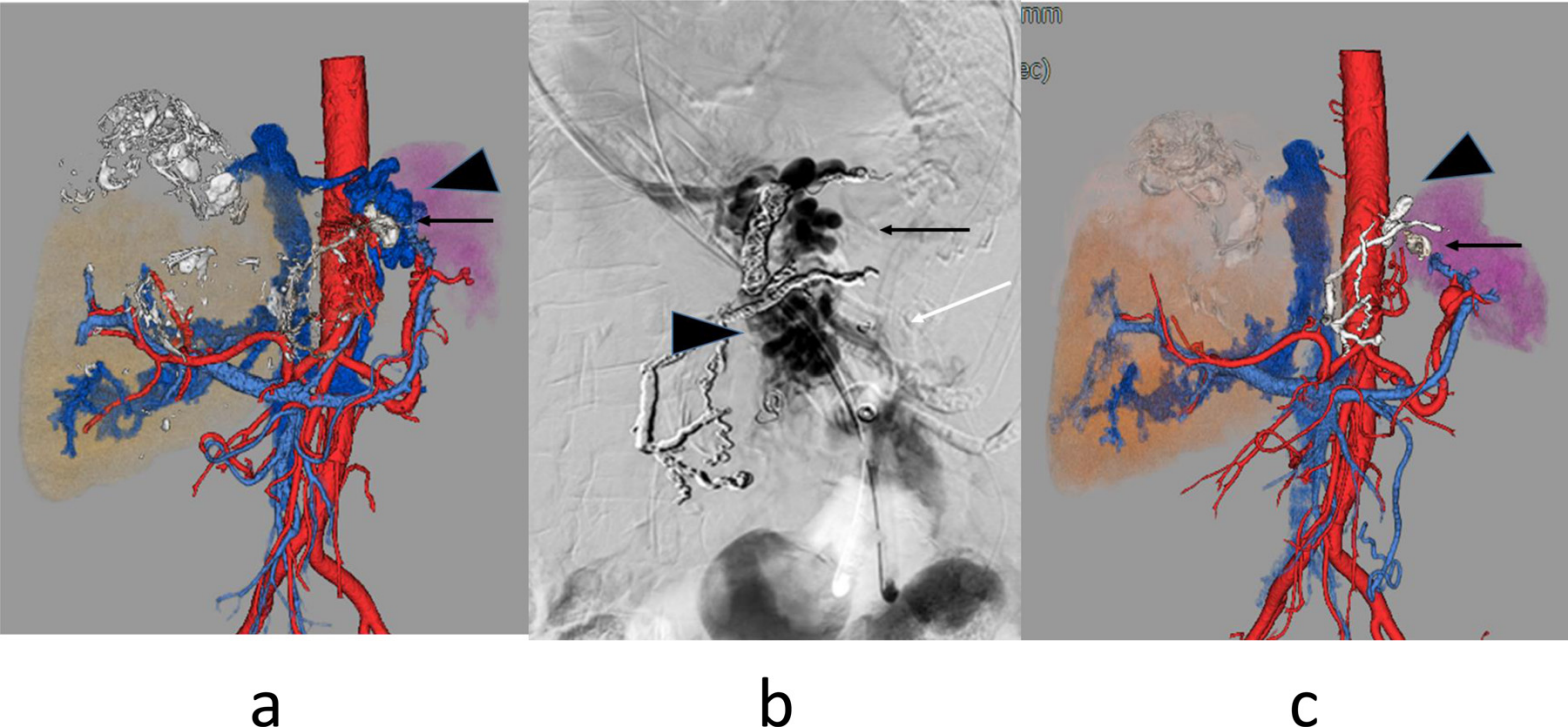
(a). 3D-CT reconstruction image after the emergency hybrid procedure in case 2 shows NBCA-Lipiodol (arrow), residual gastric varices (arrowhead), gstrorenal shunt, and inferior phrenic vein. (b). Retrograde phrenic venography during TJO shows residual gastric varices (arrow), short gastric vein (arrowhead), and peripheral splenic vein (white arrow). (c). 3D-CT reconstruction image after TJO shows NBCA-Lipiodol (arrow), embolized coils in the phrenic vein (arrowhead), no gastric varices, no gastrorenal shunt, and no phrenic vein.
